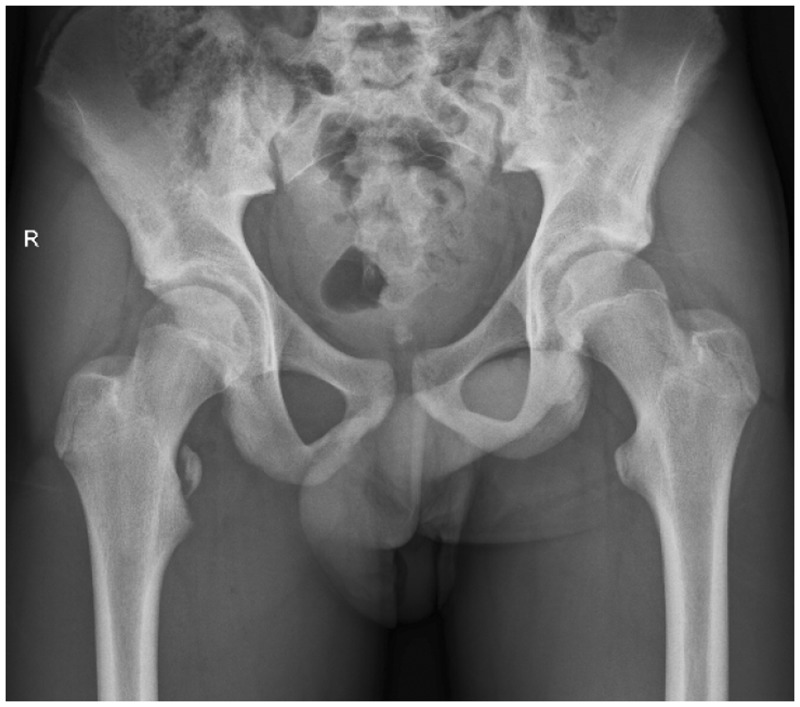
Plain radiograph showing an avulsion of the right lesser trochanter.
